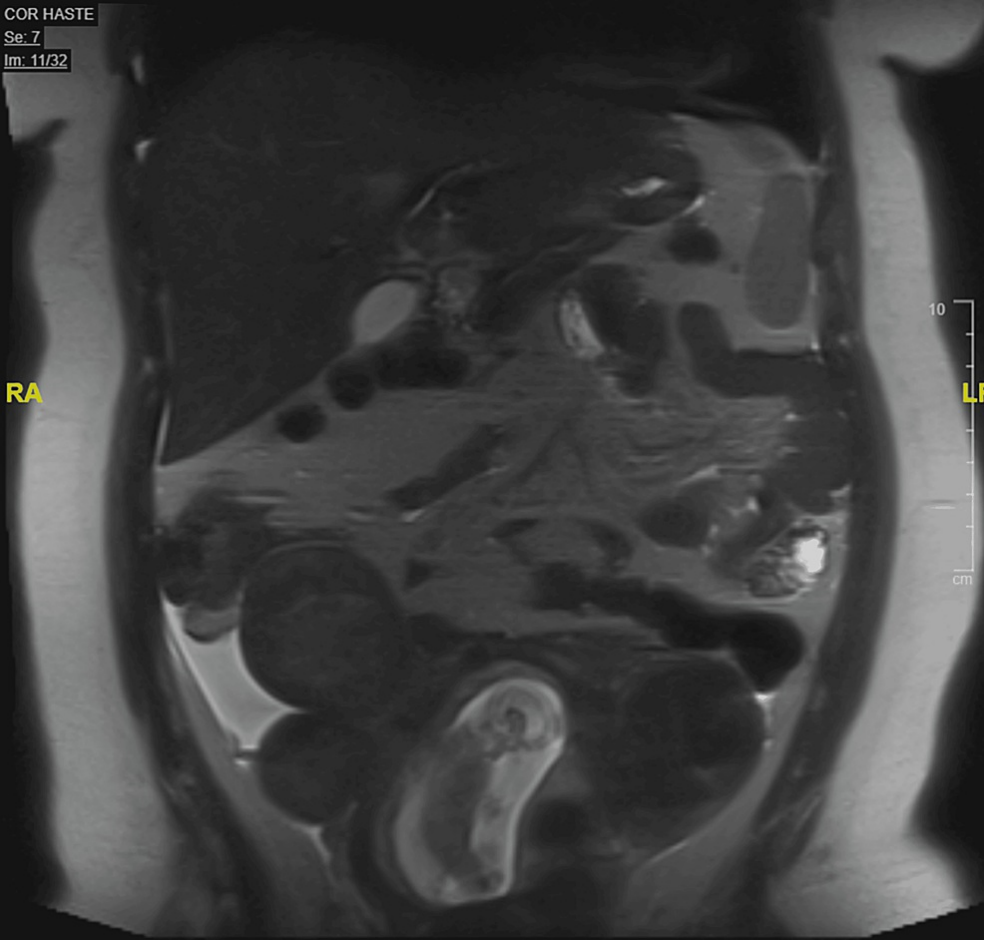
Abdominal/pelvic magnetic resonance imaging without contrast, coronal T2 (half-Fourier acquisition single-shot turbo spin echo) sequence
